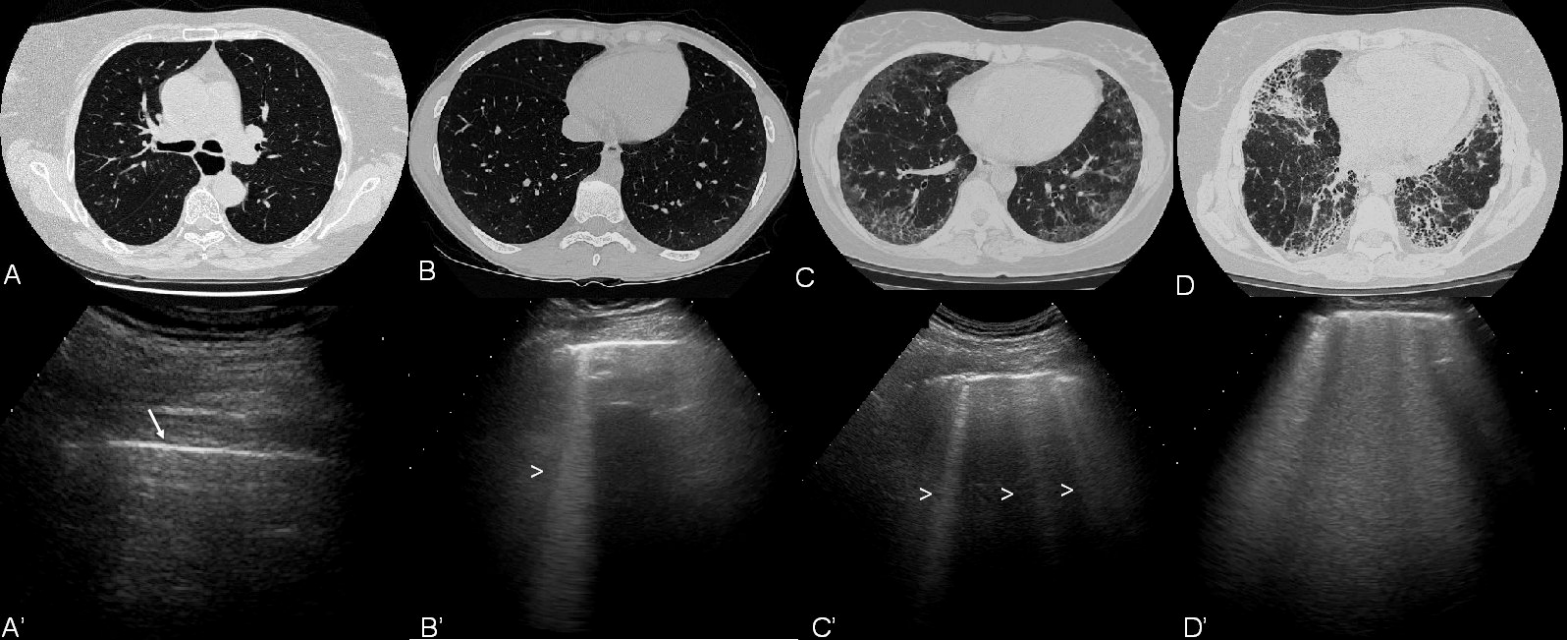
HRCT features of interstitial pulmonary fibrosis. A. Normal aspect of the lung. B. Mild. C. Moderate. D. Severe. A'. US examination of healthy interlobular septa at lung surface level. Note as the pleura is a linear and regular hyperechoic band (arrow). B'-D'. US examinations showing different scores of fibrotic pulmonary involvement: B'. Mild. C'. Moderate. D'. Severe.
